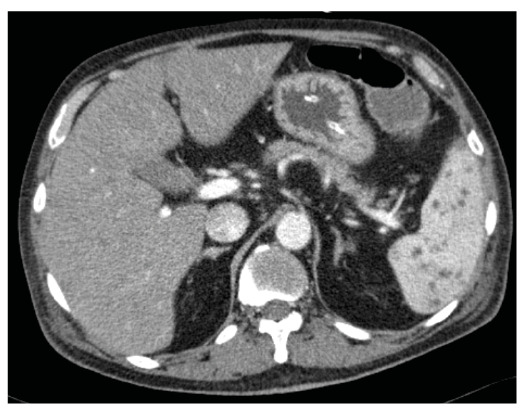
Computed tomography scan of the thorax and abdomen showing the presence of multiple splenic microabscesses.
